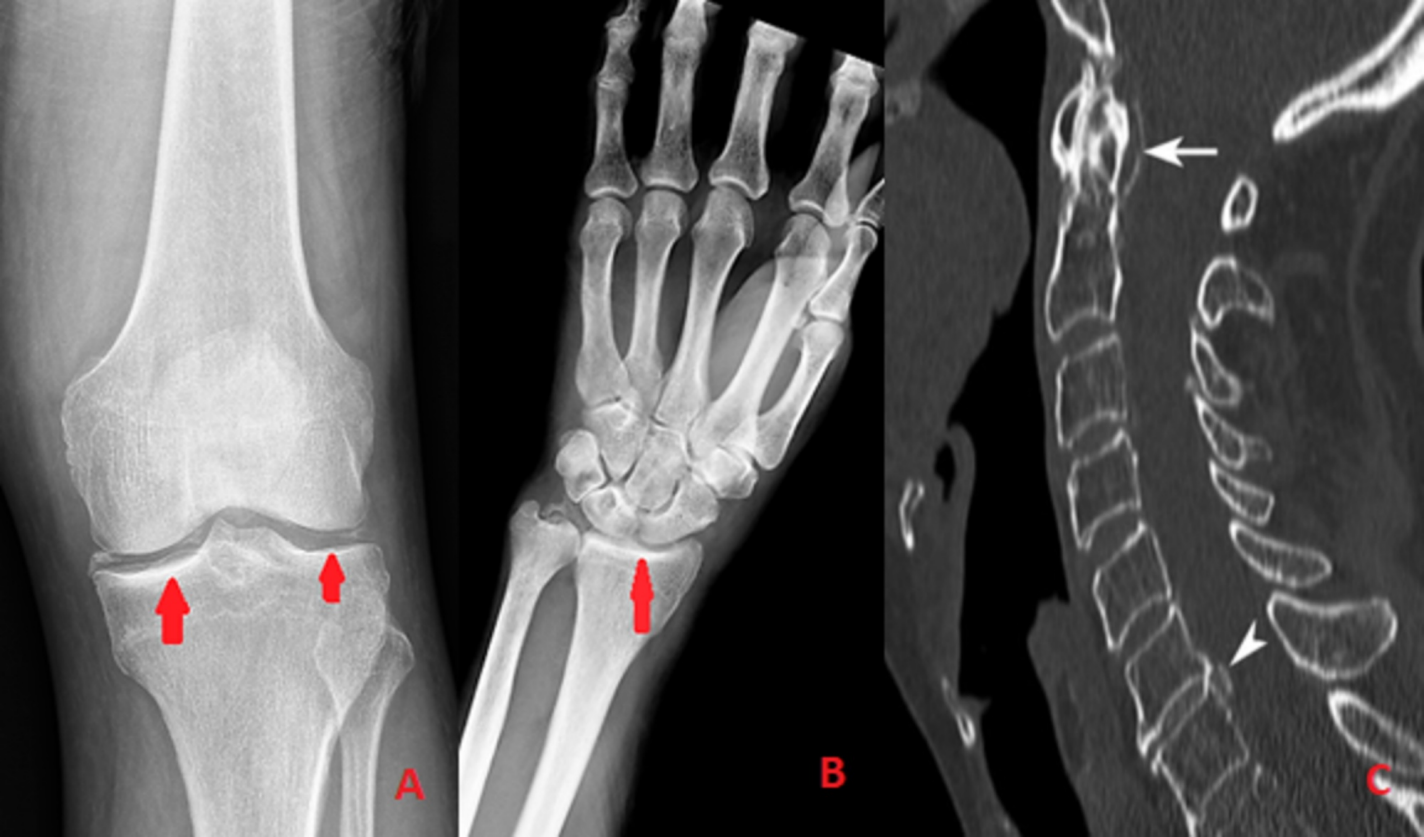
A & B showing chondrocalcinosis evident on Xray, C showing crowned dense syndrome.
